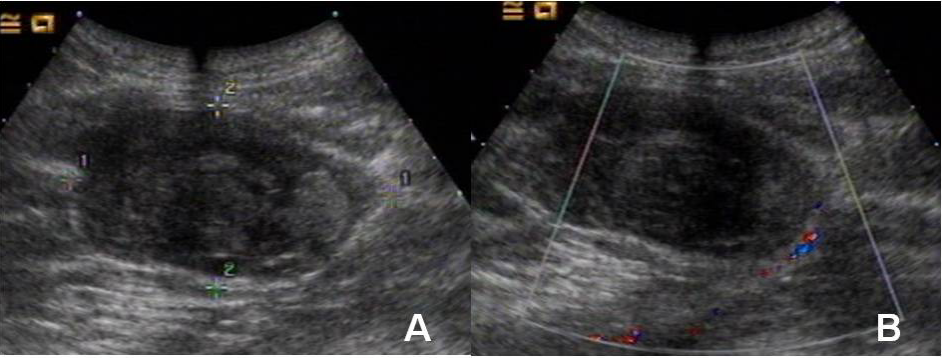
An ultrasonographic examination of the mass demonstrated lobulated low level densities in the gluteus maximus muscle (panel A). A color-flow Doppler ultrasonography was performed without abnormal color signal within the hemorrhagic mass (panel B).
